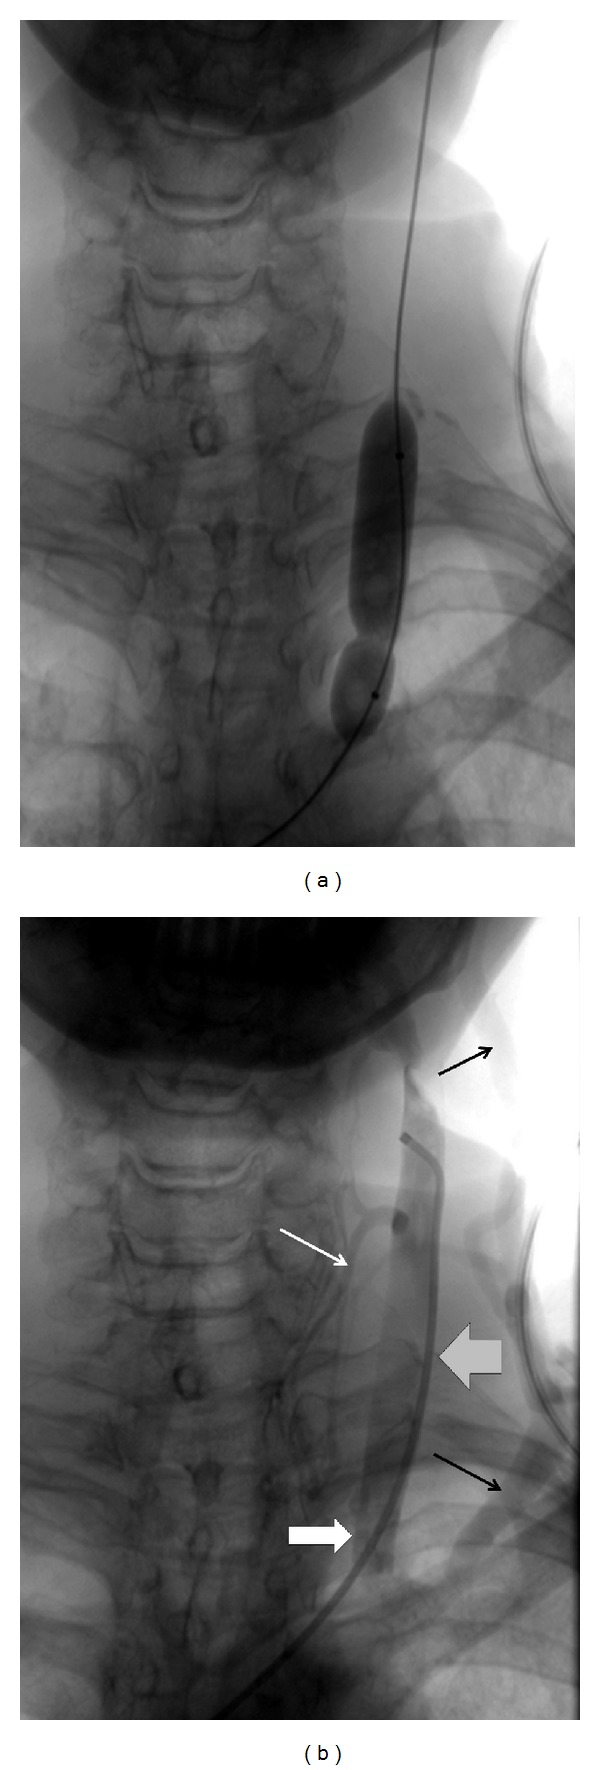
(a) Balloon angioplasty of the left internal jugular valve; impression on the balloon indicates the location of restricting annulus. (b) Post-balloon venography of the left internal jugular vein: satisfactory outflow from the vein; stenosis that was previously seen during angioplasty (thick white arrow) disappeared, there is, however, still collateral outflow through the external jugular vein (thin black arrows) and the thyroid veins (thin white arrow); thick grey arrow points the area of compression by omohyoid muscle (revealed by means of sonography at followup): this compression does not show up at venography.
